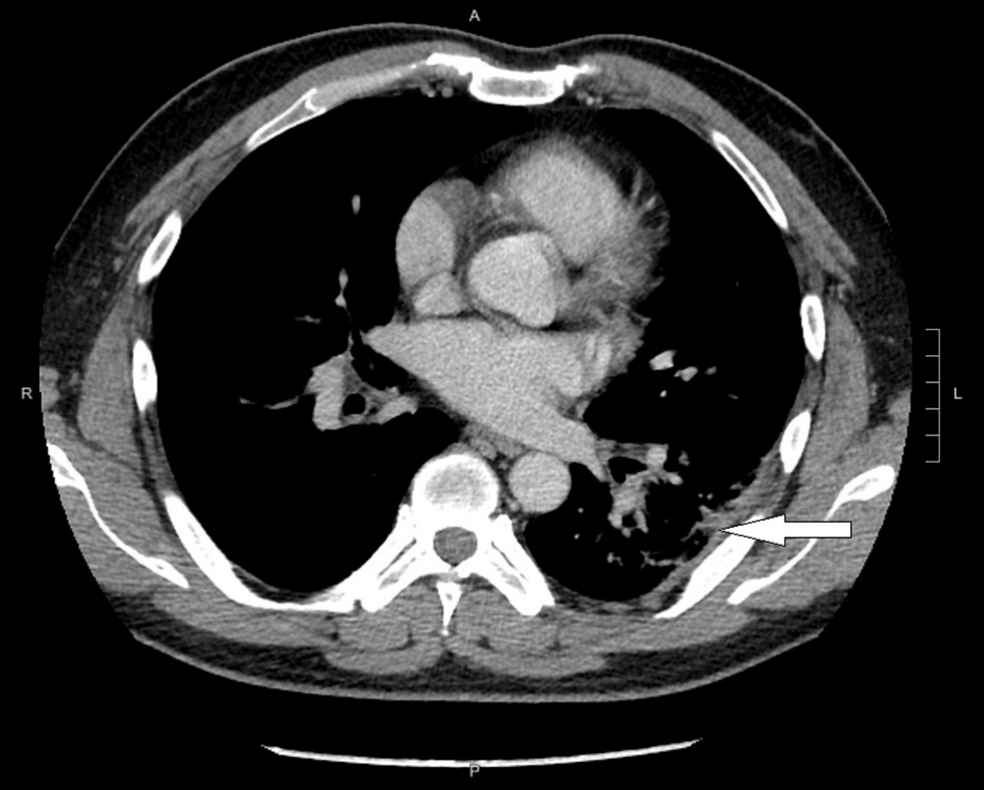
Contrast-enhanced CT image of the patient after the CA-MRSA treatment The white arrow points toward a resolved lung abscess in the left lower lobe. CA-MRSA, community-acquired methicillin-resistant *Staphylococcus aureus*
